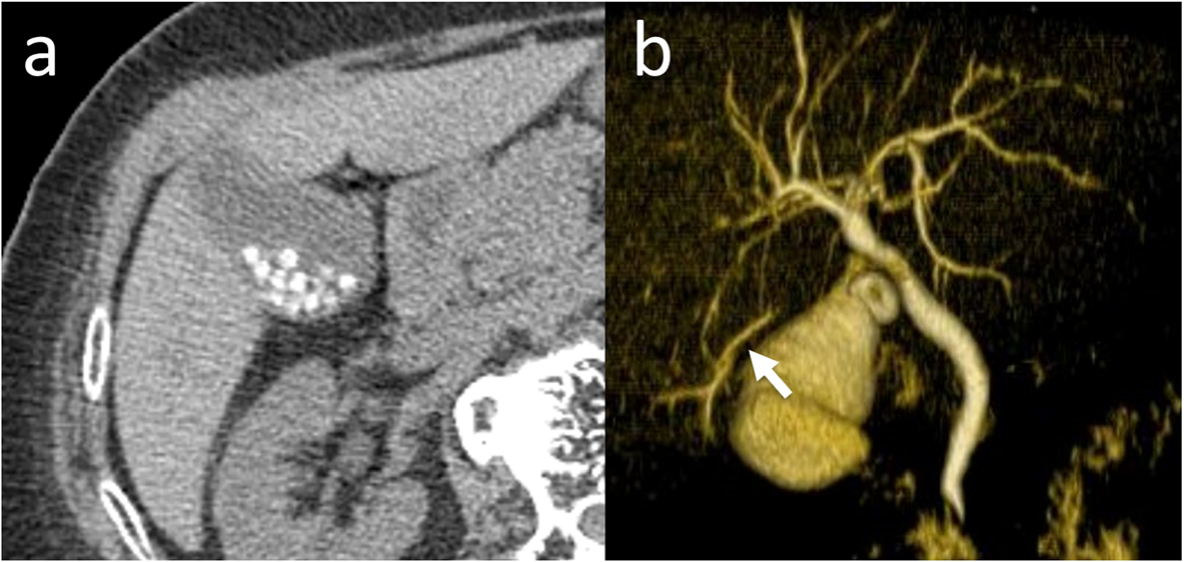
a Computed tomography scan revealing multiple calcified stones in the gallbladder. b Preoperative drip infusion cholecystocholangiography-computed tomography showing a bile duct branch with no communication between the intra- and extrabiliary systems (arrow)
